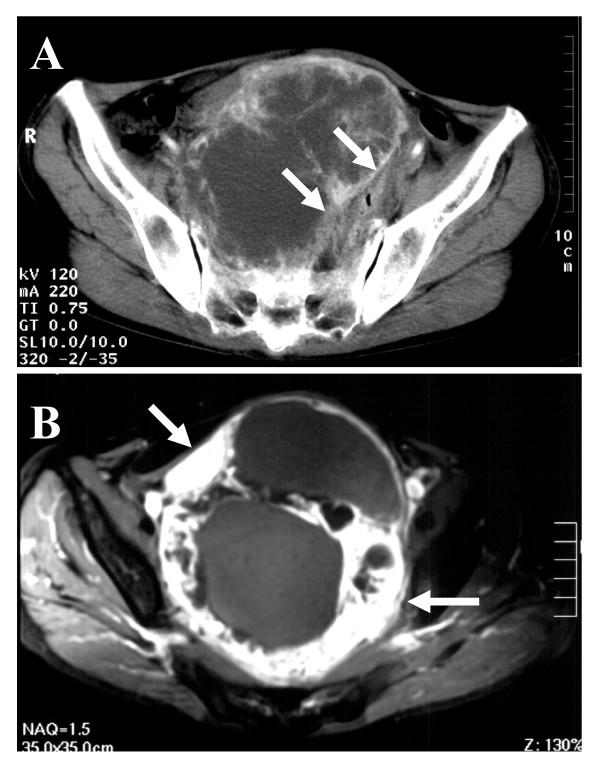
A) An abdominal CT revealed a large uneven tumor in the lower abdomen and encased the colorectum to the left back side (*arrows*). B) An MRI of the lower abdomen revealed a polycystic tumor with a part of the thickened wall (*arrows*).
